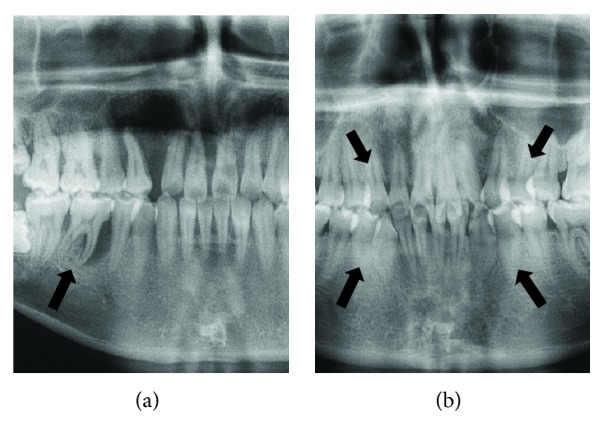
Representative intraoral dental radiographs of donors. (a) Radiograph of a donor with periodontitis shows severe periodontal loss. (b) Radiograph of a healthy donor undergoing teeth extractions for orthodontic treatment purposes. The arrows indicate the teeth that will be extracted.
